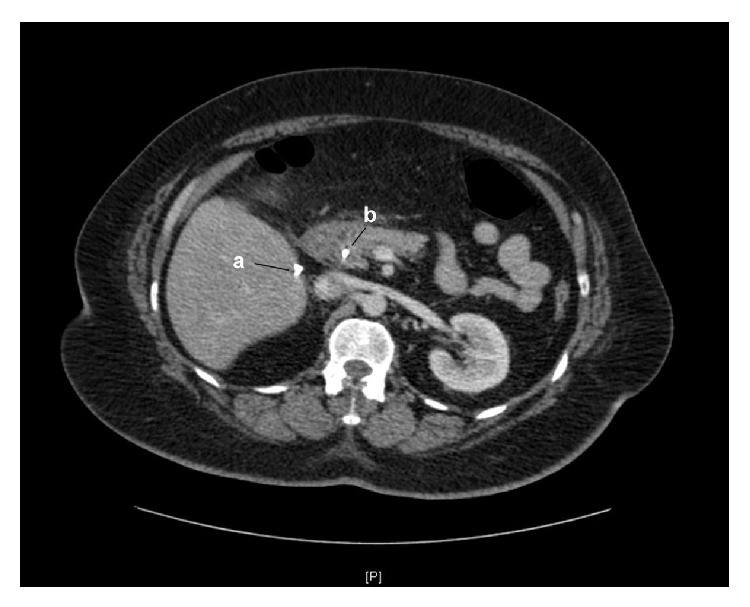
CT abdomen demonstrating (a) hyperdense material corresponding to surgical clip(s) in gallbladder fossa and (b) hyperdense material in the distal CBD.
